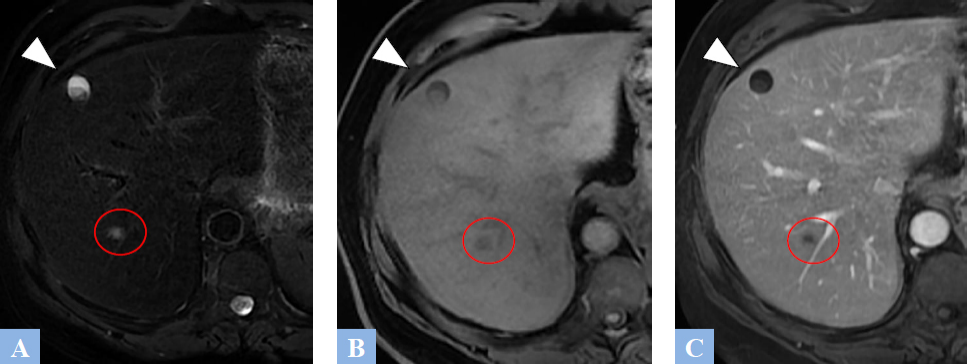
Ciliated hepatic foregut cyst in a 60-year-old male patient undergoing treatment for a metastatic colorectal cancer. A. T2-weighted fat-suppressed MR image in the axial plane showing a well-defined cystic mass in segment IV, which is markedly hyperintense on T2 (arrowhead). A more posterior metastatic lesion is noted, with poorly defined margins, exhibiting an intermediate T2 signal (indicated by the red circle). B. T1-weighted fat-suppressed MR image in the axial plane. The cystic lesion in segment IV appears hypointense, with hyperintense dependent sediment (arrowhead). The metastasis also exhibits T1 hypointensity, though it is less pronounced (red circle). C. T1-weighted fat-suppressed MR image in the axial plane after gadolinium administration at the portal phase of enhancement showing no cystic enhancement (arrowhead). The secondary lesion, in turn, shows poorly defined peripheral enhancement (red circle).
